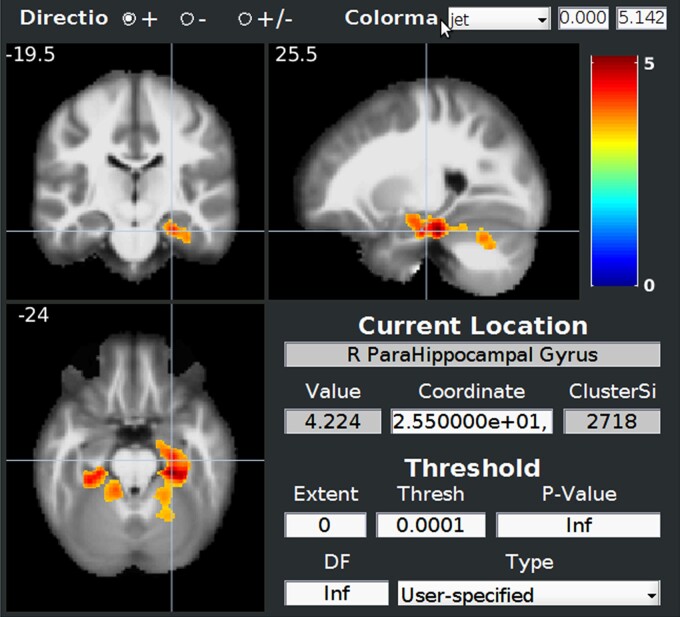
Comparison of MRI segmentation of GM between 18 DM1 patients and 18 controls showing mostly parahippocampus involvement. Normalized data were smoothed with an isotropic Gaussian kernel of 8 mm. Statistical analysis was performed using a general linear model with age, sex and total intracranial volume as covariates. Statistics were corrected for multiple comparisons with FWE correction at the cluster level with a height threshold of 0.001. The statistical map is showed through BSPM view.
